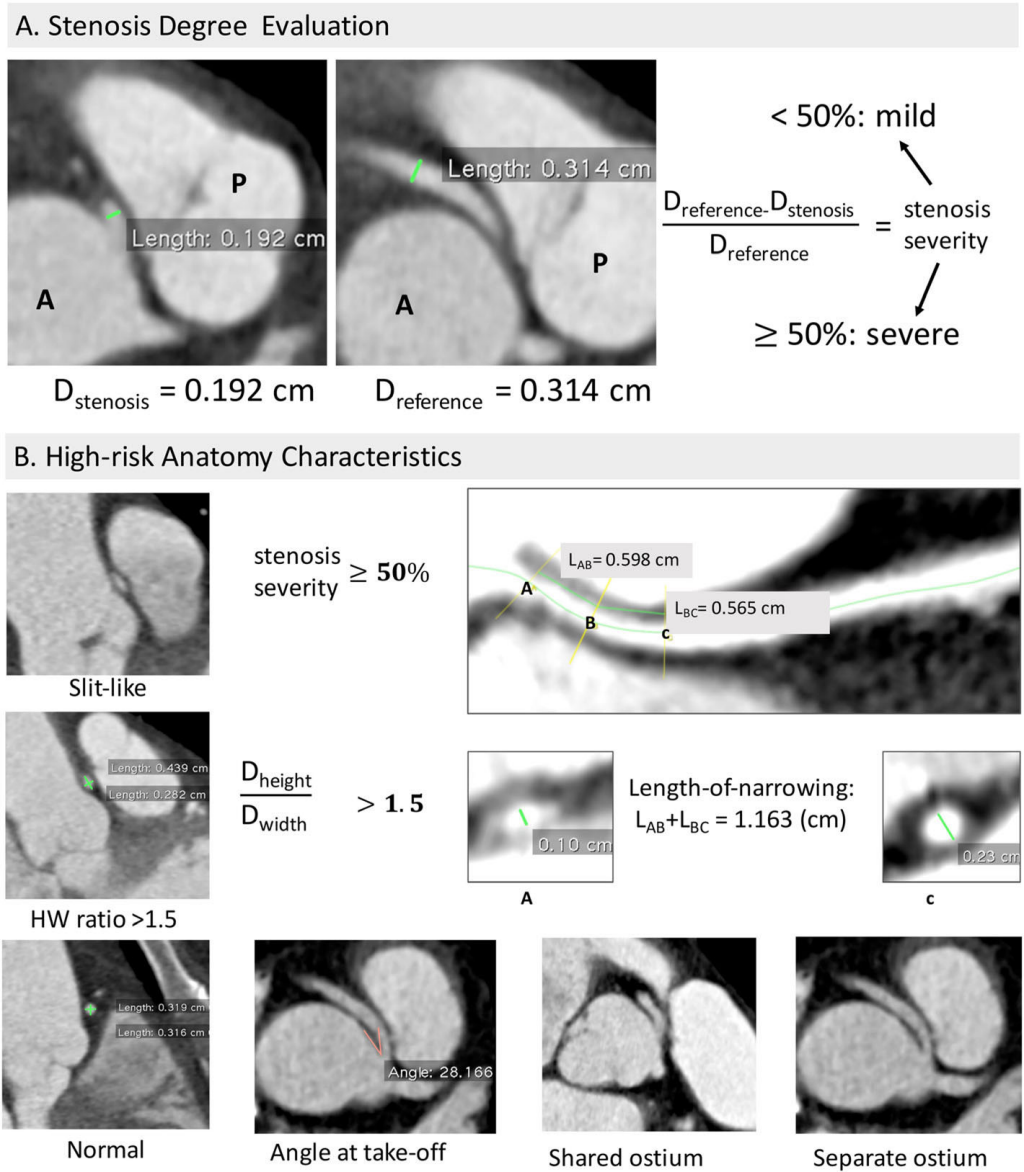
CTA image analysis protocol for the ACAOS. a For stenosis severity ranking, a degree of stenosis less than 50% were classified as mild stenosis and a degree of stenosis equal or more than 50% were classified as severe stenosis. b the high-risk anatomy characteristics identification protocol, including “slit-like” vessel, Height-to-width ratio (HW ratio), angle at the take-off level, and the shared or separate ostium
